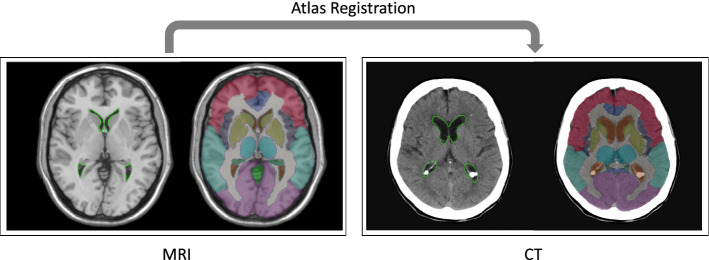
Exemplary registration result: Overlay of only the ventricle label and all anatomical labels on MRI atlas (left) and CT volume (right)
